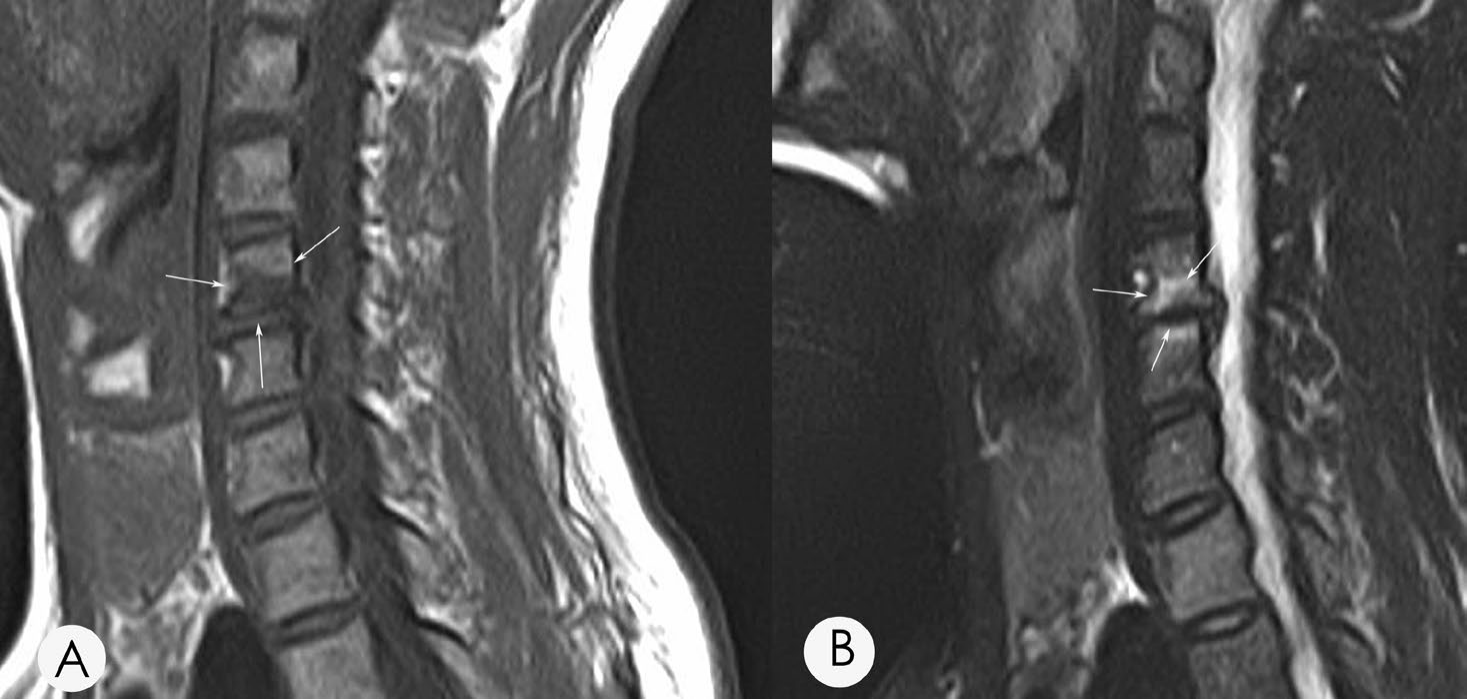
(A) A T1‐weighted magnetic resonance imaging (MRI) of a 54‐year‐old female demonstrates hypointensity at the inferior endplate of C5. (B) A T2‐weighted MRI of the same patients demonstrates hyperintensity, suggesting a type 1 MC. Arrows indicate the location of the identified MC lesion on T1 and T2 imaging.
